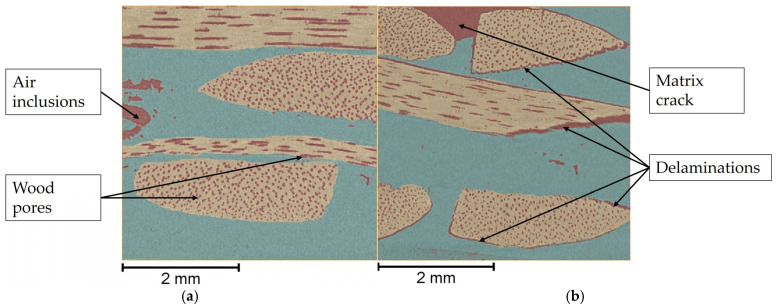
CT images of WTC after storage (a) in a standard climate and (b) after weathering.
